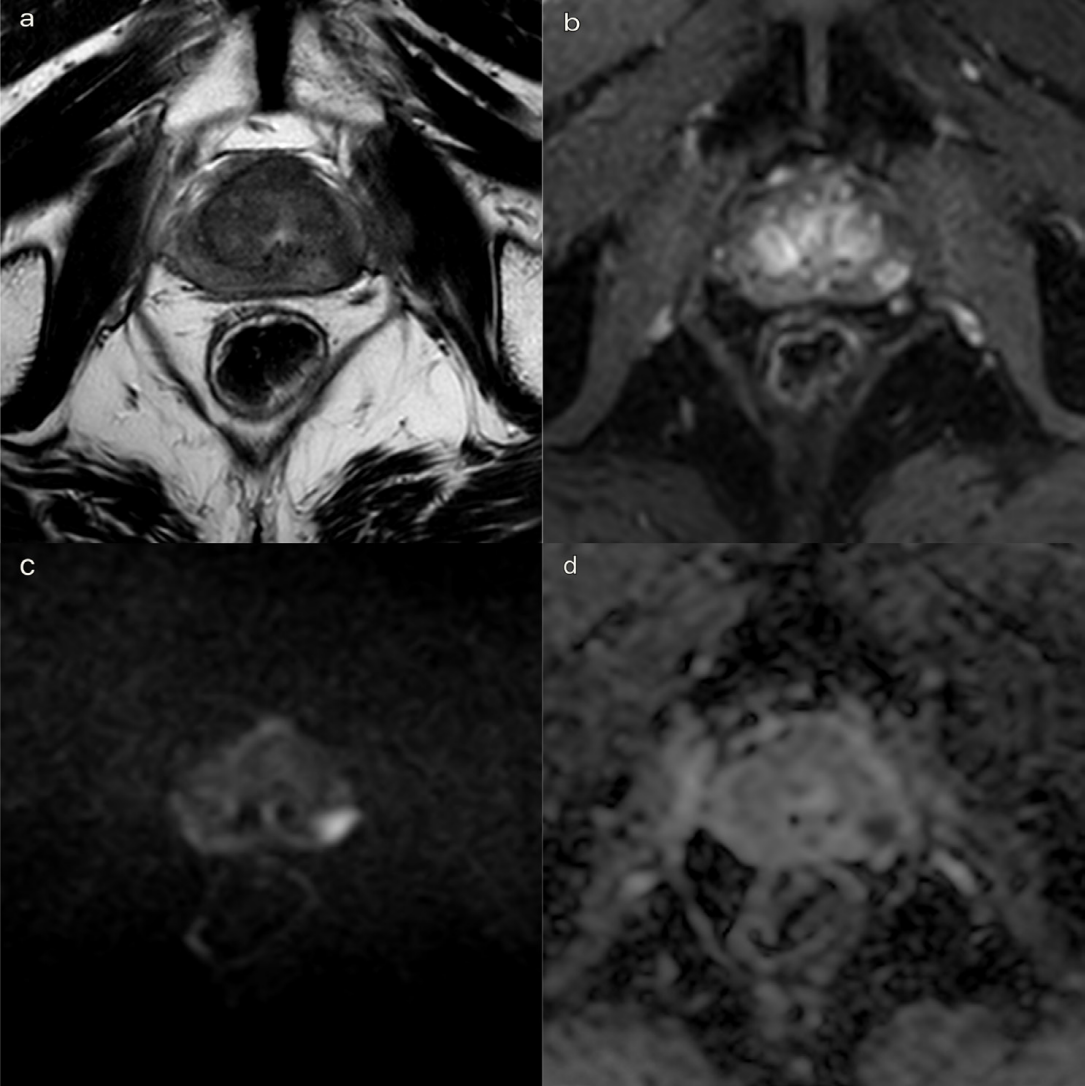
High field (3T) multiparametric MR before treatment. Focal recurrence of prostate cancer. Ultrasound/MRI-guided biopsy was performed with a Gleason score 3 + 4. (a) *T*
